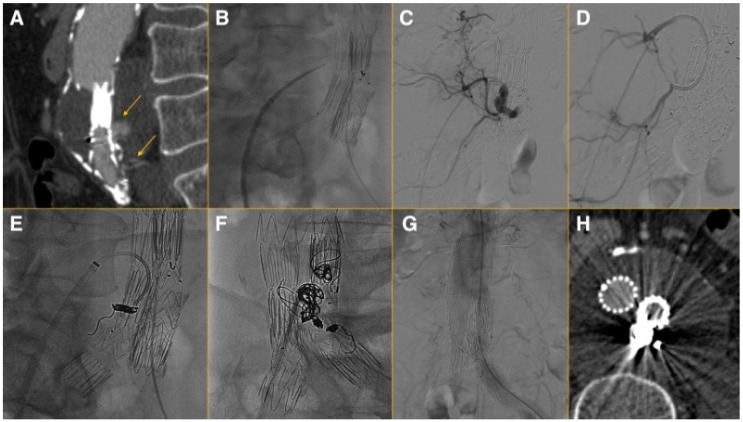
After evaluating the CTA and confirming the presence of an EL 2, especially related to the lumbar arteries (A) and if the patient presented with the anatomic criteria, they were considered to be a candidate for TCE under local or general anesthesia. Percutaneous arterial access from the right or left femoral artery was used for angiography to rule out other endoleaks. The right common femoral vein was used for venous access. An 8F SL0 sheath dilator system (St. Jude Medical, St. Paul, MN, USA) was positioned against the vena cava wall. A Brockenbrough needle (Medtronic, Minneapolis, MN, USA) was coaxially introduced and the aneurysm sac was then punctured (B). The aneurysm sac puncture site was near the endoleak nidus or contributing vessel (C). When successful puncture was confirmed, the dilator tip was forced into the sac and the SL0 sheath was exchanged for an 8F 30 cm Flexor sheath (Cook Medical, Bloomington, Ind) and a 5 Fr 65 mm catheter, which was used for the catheterization of the contributing vessel (D). Selective coil embolization of the contributing vessel was performed (E) and was followed by non-selective embolization of the nidus (F). After embolization, a final angiography was performed (G). Venous access sites were closed by manual compression and arterial access sites with either manual compression or a closure device. A pre-discharged CTA was performed to confirm EL 2 exclusion and to use as a baseline for further diameter follow-up (H).
